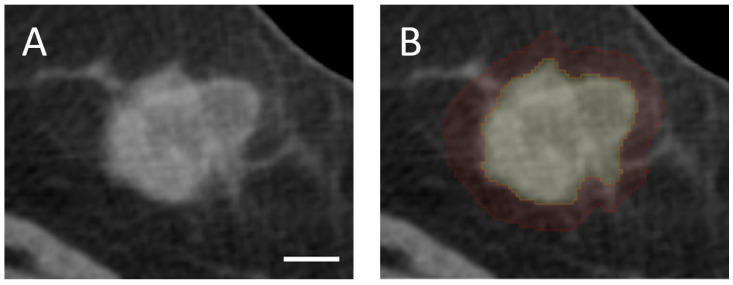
Example of tumor segmentations on pre-treatment contrast-enhanced CT images. (A) Contrast-enhanced CT image; (B) the same CT image with intratumoral (yellow) and peritumoral (red) segmentations. A scale bar corresponding to 1 cm is shown in the bottom right corner of image (A).
